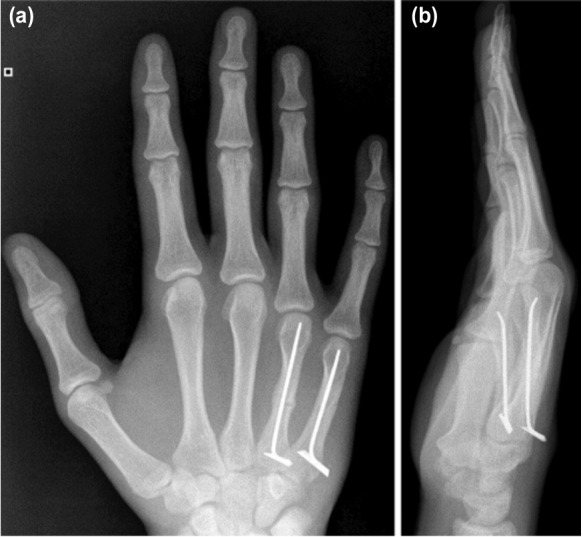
Post-operative radiographs (a) PA and (b) Lateral views of united transverse mid metacarpal ring and little finger shaft fractures with the locked intramedullary nail in-situ.
